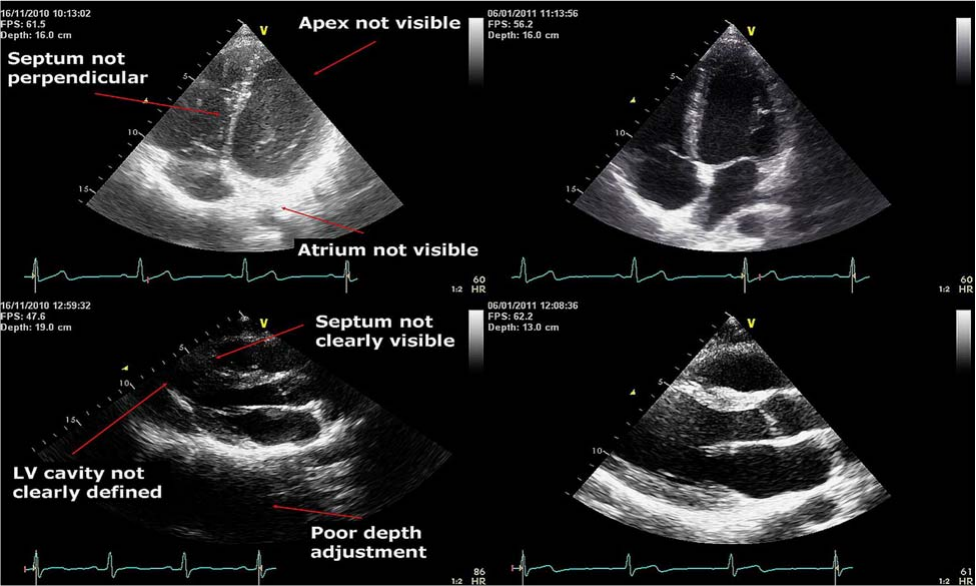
Actual examples of image quality before and after intervention.* Upper left panel:* Apical 4-chamber view at baseline. *Upper right panel:* Apical 4-chamber view at evaluation. *Lower left panel:* Parasternal long axis view at baseline. *Lower right panel:* Parasternal long axis view at evaluation.
